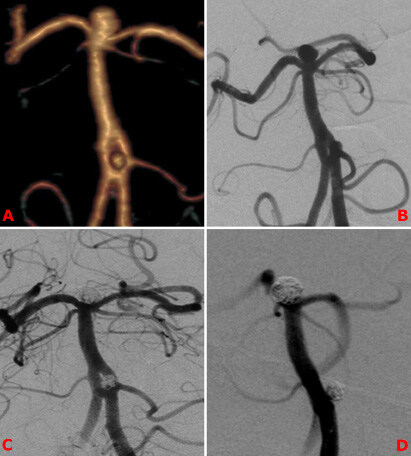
Sixty-two-year-old male patient presented with SAH. CTA and DSA show proximal BAF and relevant aneurysm. A basilar tip aneurysm is also seen (A, B). Both aneurysms have been treated with standard coiling (C). DSA control after 6 months (D).
